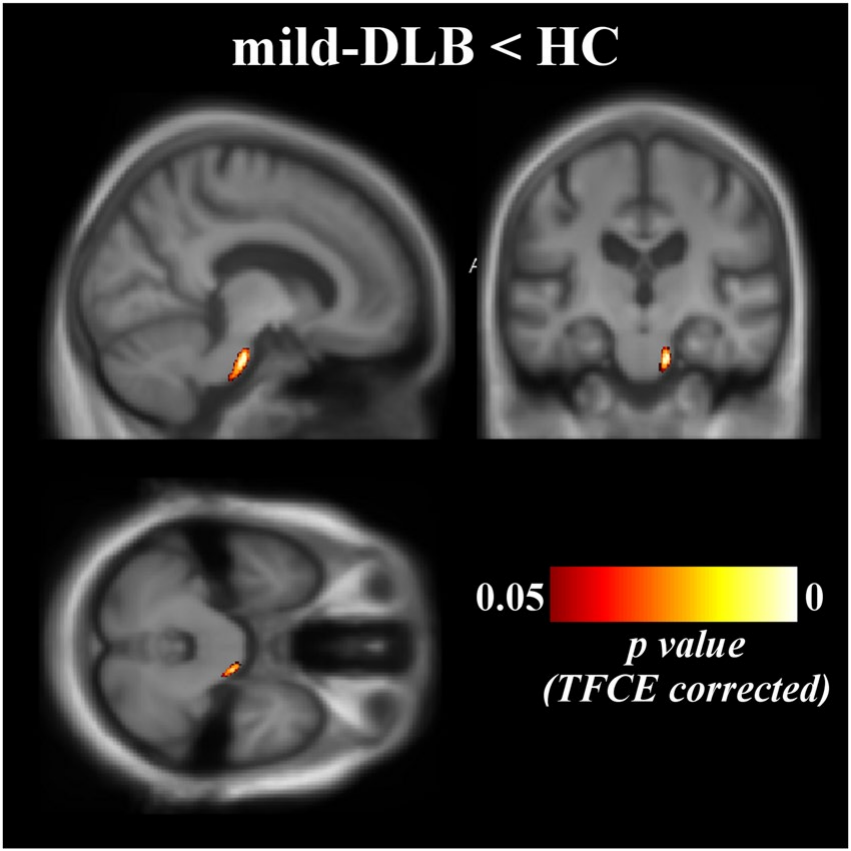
Patterns of significant white matter loss in mild-DLB patients mild-DLB, dementia with Lewy bodies at the stage of dementia; HC, healthy controls. Results are expressed as *p*-value from permutation tests with threshold-free cluster enhancement corrected for multiple comparisons, and are superimposed on the mean MNI-standardized MRI T1-weighted image of the patients at the stage of dementia and healthy controls. Left is left side of the brain. Coordinates of the multiplanar image in the MNI space: x = 11; y = −18; z = −30.
